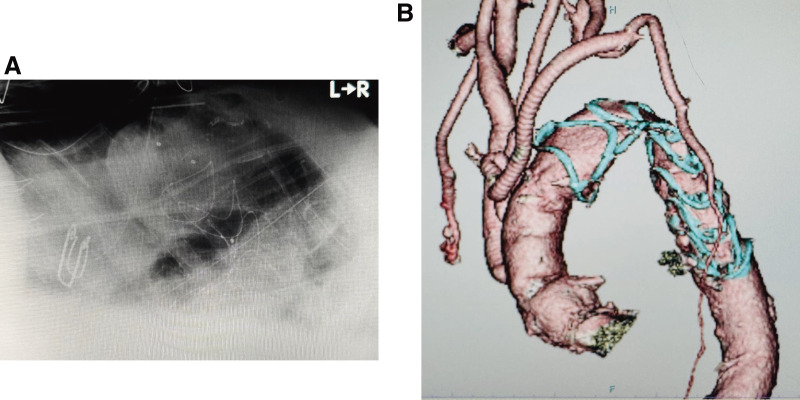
Early postoperative imaging. (A) A postoperative chest radiograph indicating potential kinking and stenosis of the arch stent graft. (B) A contrast-enhanced CT scan from POD 1, confirming severe stenosis of the Thoraflex Hybrid prosthesis (Terumo Aortic, Inchinnan, UK) in the aortic arch. CT: computed tomography; POD: postoperative day
